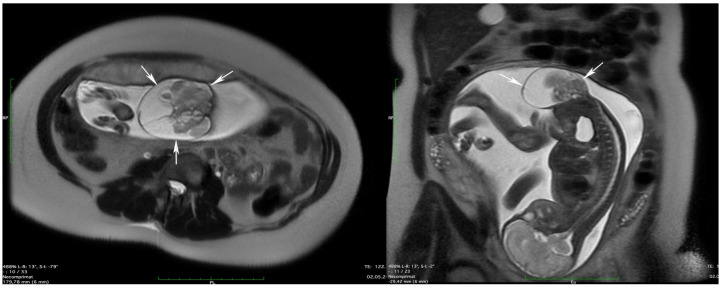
Fetal MRI. Note the type I sacrococcygeal teratoma—transversal and sagittal T2-weighted (white arrows).
